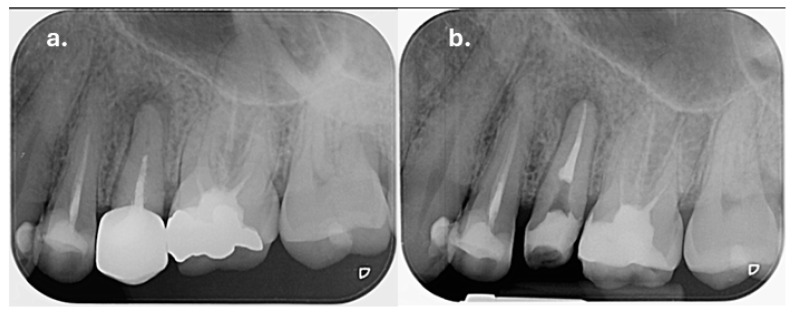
Preoperative radiograph of tooth 25: (a) showing the diffuse radiolucency due to the periapical inflammation; (b) immediately after root canal obturation.
